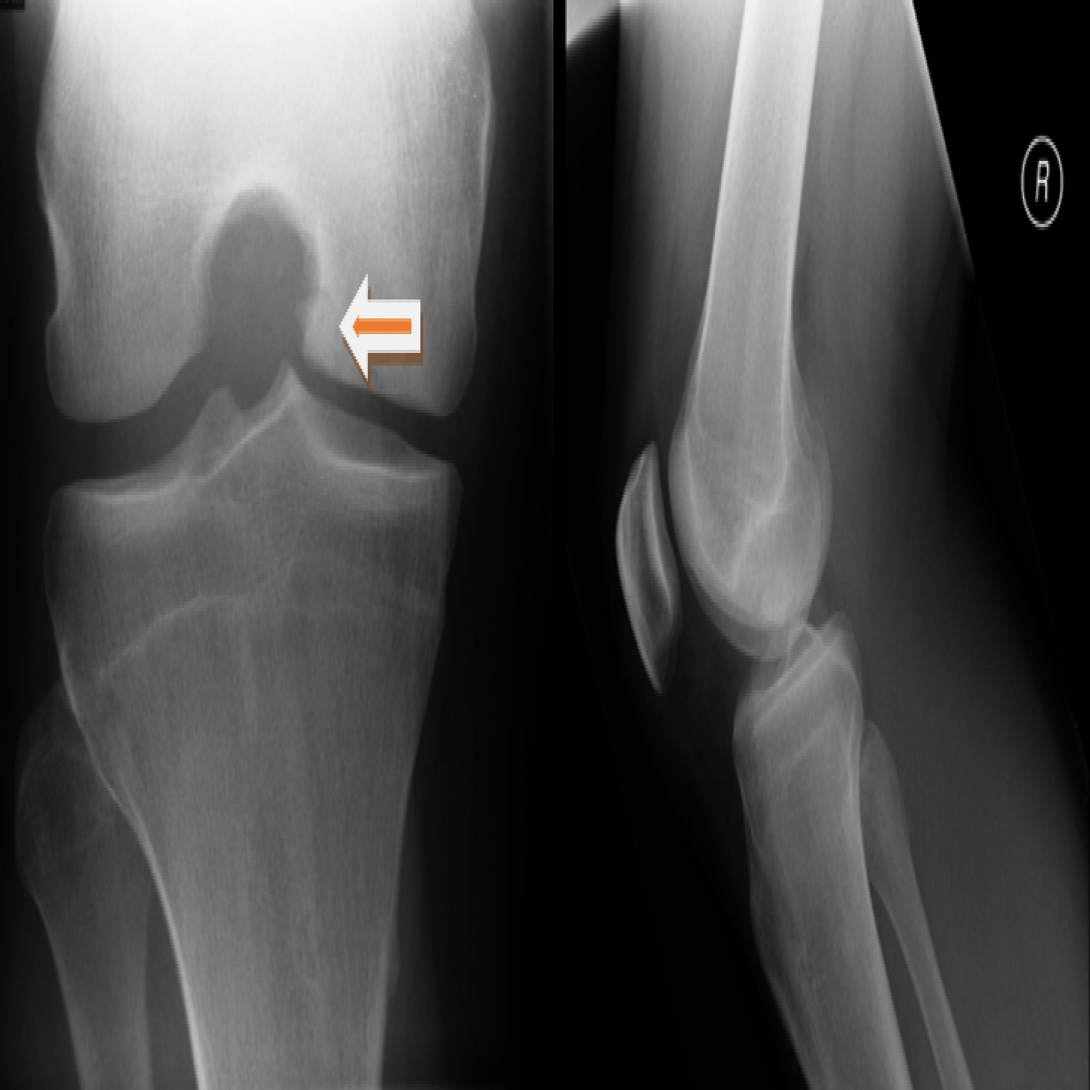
Radiograph showing Osteochondral lesion
